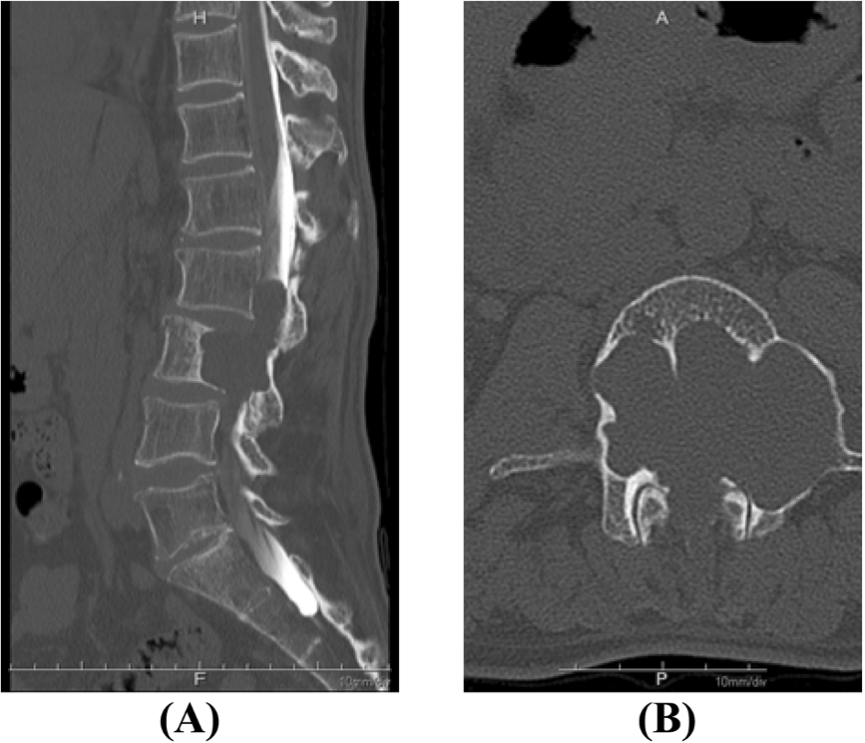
Large scalloping of the L3 vertebral body. Pre-operative sagittal (A) and axial (B) computed tomography myelography scans of the lumbar spine show extremely extensive scalloping of the L3 vertebral body.
